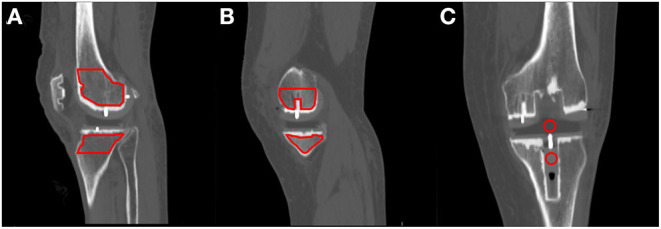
The CT density measurement method. In the sagittal position, the lateral and medial condyles were selected centrally (midpoint of the metal alignment line), and the cancellous area was encircled using the Weasis polygon tool (A,B). The HU values of the HXLPE bearing and the PEEK tibial prosthesis stem (stem) were selected in the coronal position (C). The ROI is distributed up to the highest area of the femoral prosthesis to the joint line a, and the lowest area of the tibial plateau is visible on CT to the joint line. CT, computed tomography; HU, Hounsfield unit; HXLPE, highly cross-linked polyethylene; PEEK, polyetheretherketone; ROI, region-of-interest.
